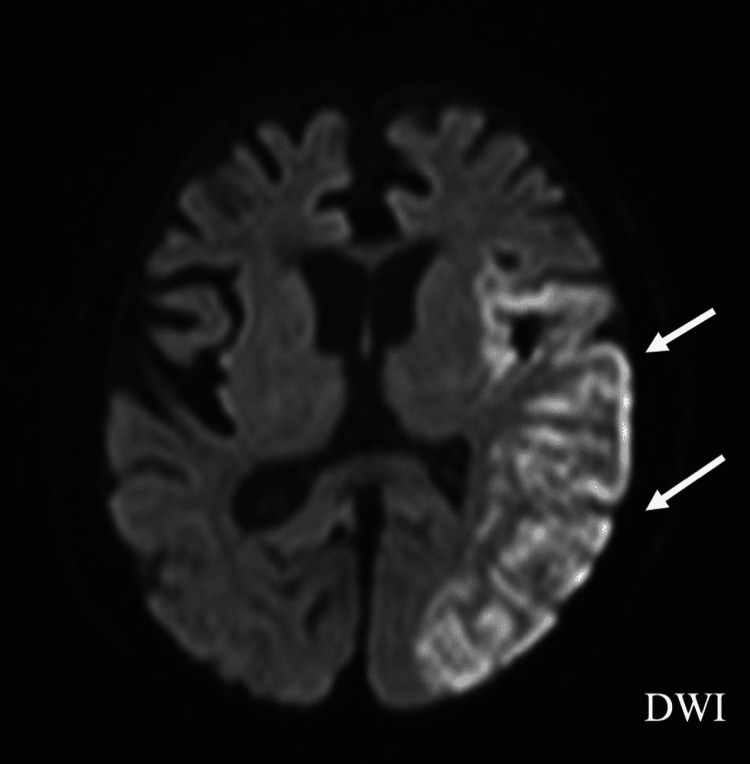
Diffusion-weighted MRI Diffusion-weighted brain magnetic resonance imaging (MRI) demonstrating extensive acute cerebral infarction involving the left hemisphere (arrow) DWI: diffusion-weighted imaging
